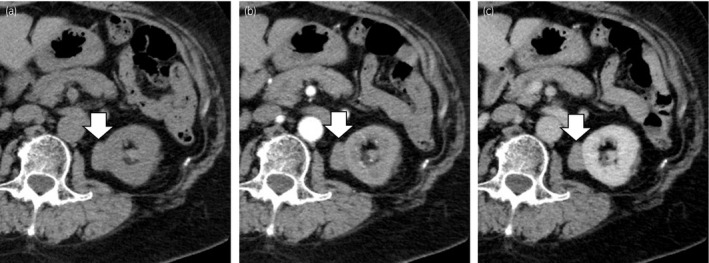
Enhanced abdominal CT shows a slowly and weakly enhancing mass 39 × 17 mm in diameter in the left kidney; (a) plain, (b) early phase, (c) late phase. Arrows: tumor.
